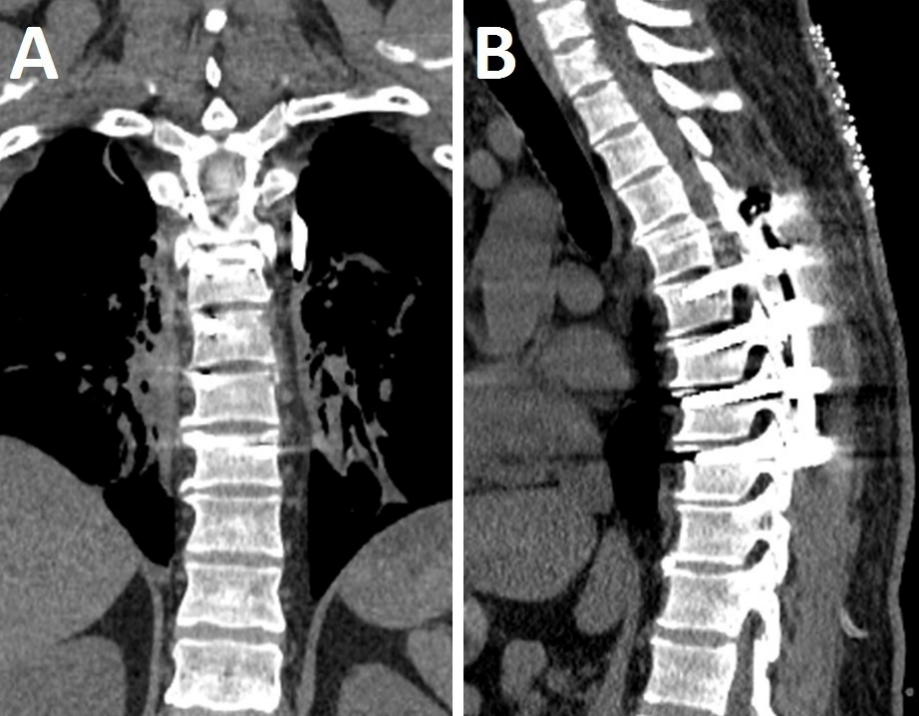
Postoperative CT Imaging Postoperative coronal (A) and sagittal (B) CT images showing postoperative changes consistent with recent T5-T9 posterior spinal fusion. There is no evidence of hardware fracture or loosening.
